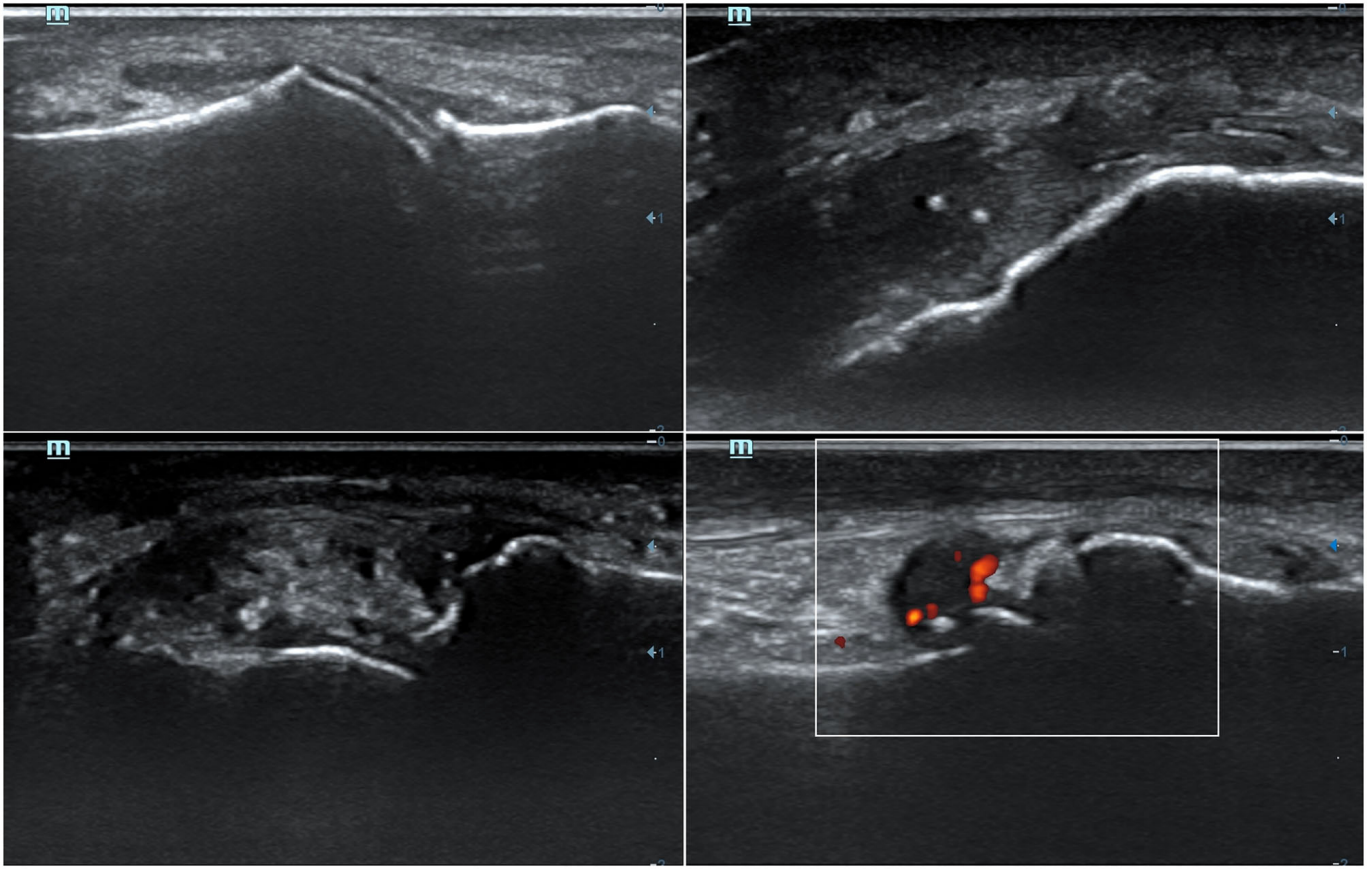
Representative ultrasound features of monosodium urate crystal deposits and associated inflammation. (Up-left) A double contour sign at the cartilage of the 1st metatarsal bone. (Up-right) Two aggregates inside distal patellar tendon. (Bottom-left) A tophus seen at the radial aspect of the 2nd metacarpophalangeal joint. (Bottom-right) A positive (grade 2) power-Doppler signal at 1st metatarsophalangeal joint, indicative of synovitis.
